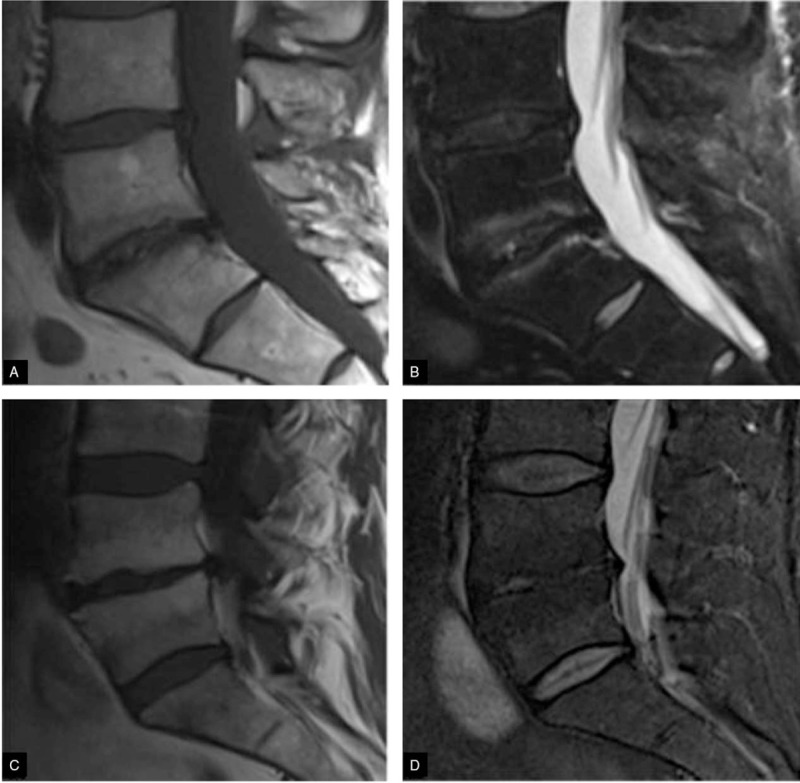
MR images of lumbar disc at the different levels from 1 to 4. The findings at the different levels 1 to 4 are: A At the level of the disc, there is minimal spinal canal narrowing by bulging of the disc and facet arthrosis. (B) At this level, there is severe spinal canal stenosis due to bulging disc and facet arthrosis. There is also an annular tear (high signal) through which the disc herniates (yellow arrow) (C) At the level of the lateral recess, there is a focal herniation of disc material compressing the L5 nerve (yellow arrow). This is called an extrusion because the distance between the edges of the disc material is greater than the distance at the base. “And (D) Compressed” L5 nerve (blue arrow) within the lateral recess. The herniated disc has migrated caudally and is seen as an oval structure anterior to the nerve. The herniated disc is uncontained, i.e. not covered by fibers of the annulus.
